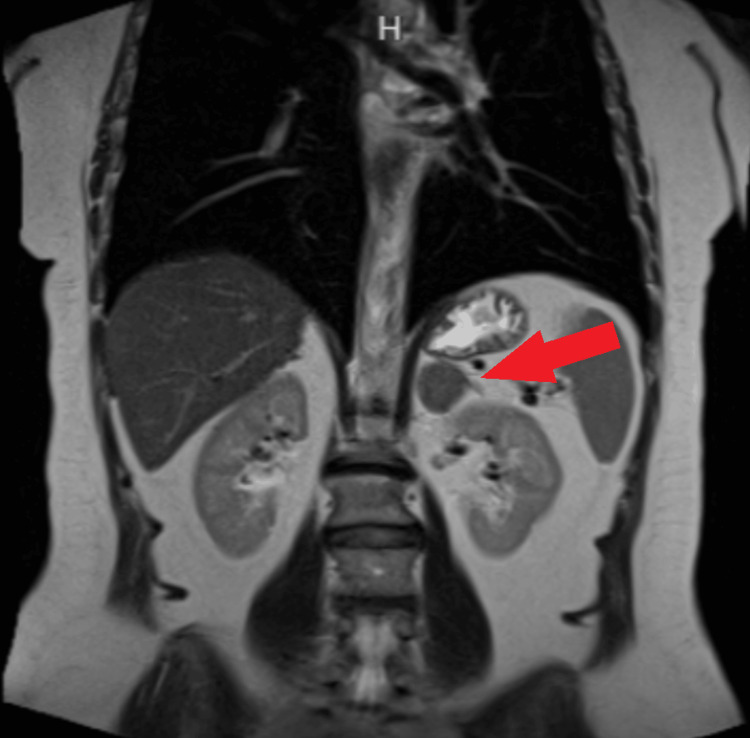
Magnetic resonance imaging (MRI) of the abdomen showing the patient’s left adrenal mass
